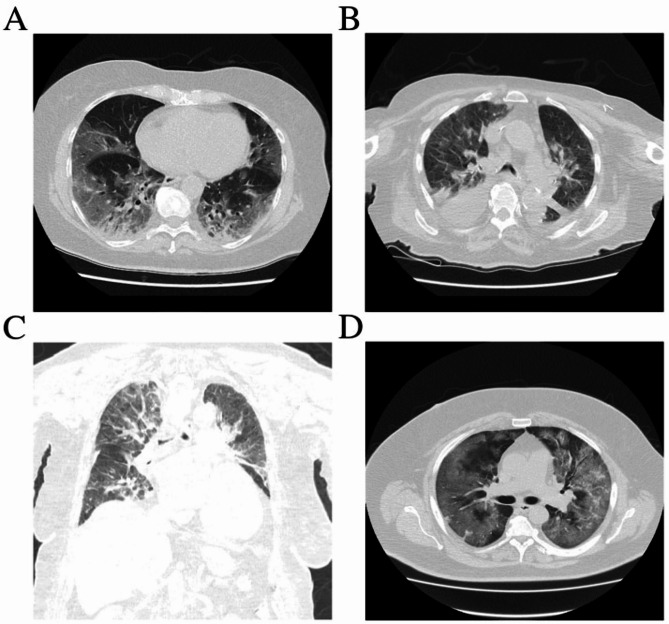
Noncontrast axial and coronal chest CT images showing inflammatory lung changes in hospitalized COVID-19 patients. (A) Extensive ground-glass opacities with consolidation, primarily in the lower lobes; ~50% of the lung parenchyma affected. (B) Bilateral ground-glass opacities with marked interlobular septal thickening, subsegmental atelectasis or consolidation, bilateral pleural effusions (5 cm right, 2 cm left), and mediastinal lymphadenopathy (16 × 11 mm); ~90% parenchymal involvement. (C, D) Confluent ground-glass opacities and dense consolidations involving all lobes, most prominent in the left upper lobe; ~70–75% right and 75–80% left lung parenchyma affected.
